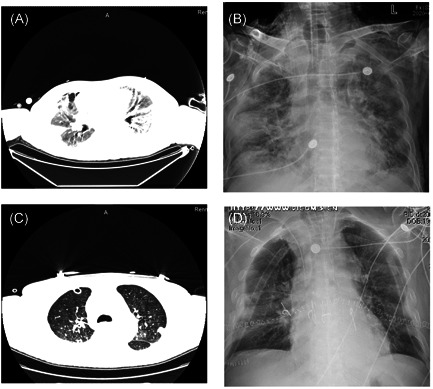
Chest CT and X‐ray image of patients. (A, B) The patient's chest CT before lung transplantation. (C, D) The patient's chest X‐ray on the fifth day after lung transplantation. CT, computed tomography
